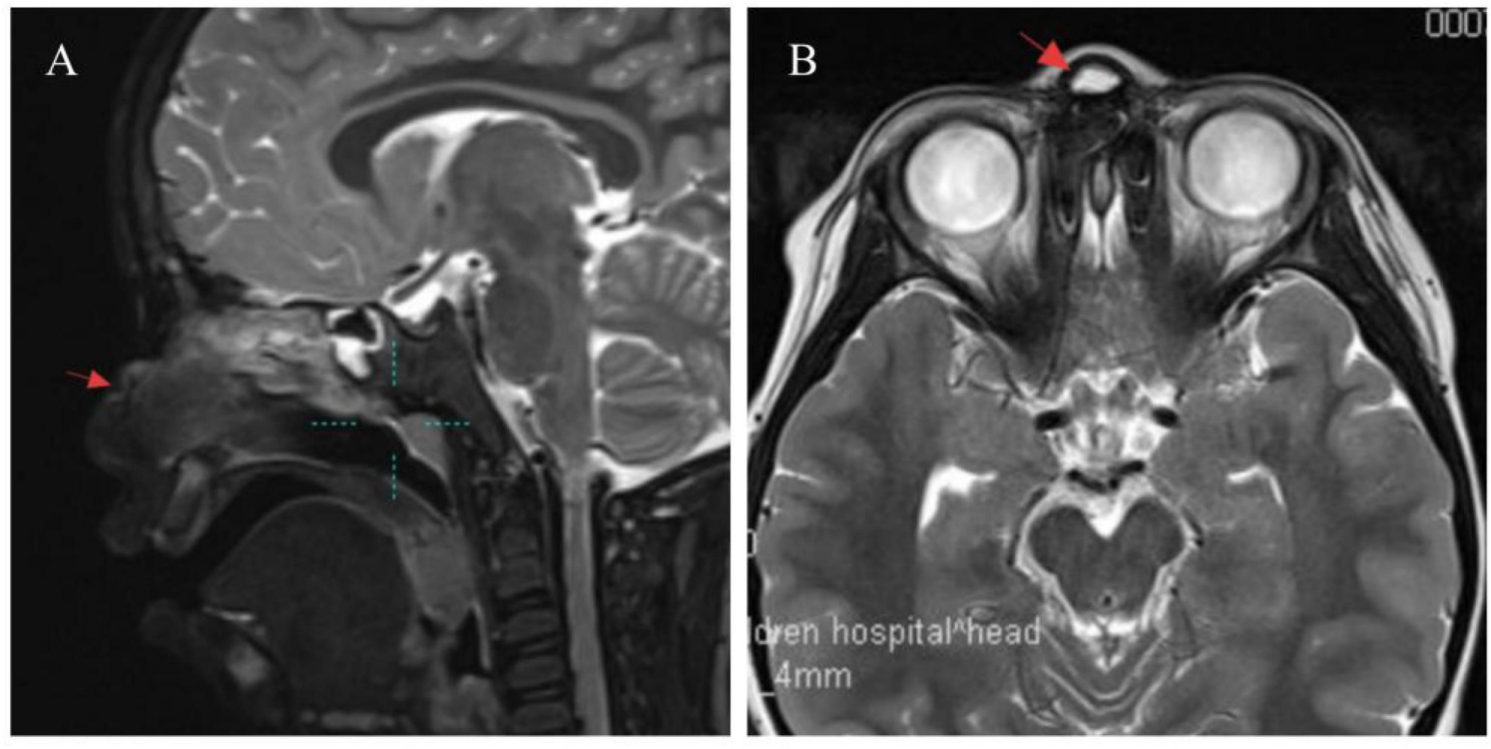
Representative MRI image of nasal dermoid sinus cyst in pediatric. (A) The area indicated by the arrow is a nodular long T1 and long T2 signal at the root of the nose. The mass is connected to the anterior skin and no communication with the intracranial cavity is seen. The posterior wall bone tissue is slightly compressed. (B) The tumor indicated by the arrow shows a high signal of T2 lipids and has a clear boundary.
